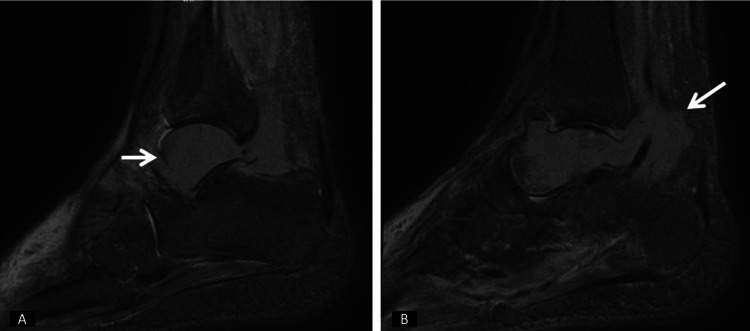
Sagittal MRI of the left ankle, obtained with T2-weighted sequences following contrast administration, shows abnormal signal intensity and contrast enhancement in the distal tibia, talus, and surrounding soft tissues, initially suggestive of osteomyelitis. (A) demonstrates involvement around the talus, while (B) highlights involvement in the soft tissue surrounding the talus. The white arrows indicate the area of the lesion. MRI: magnetic resonance imaging
